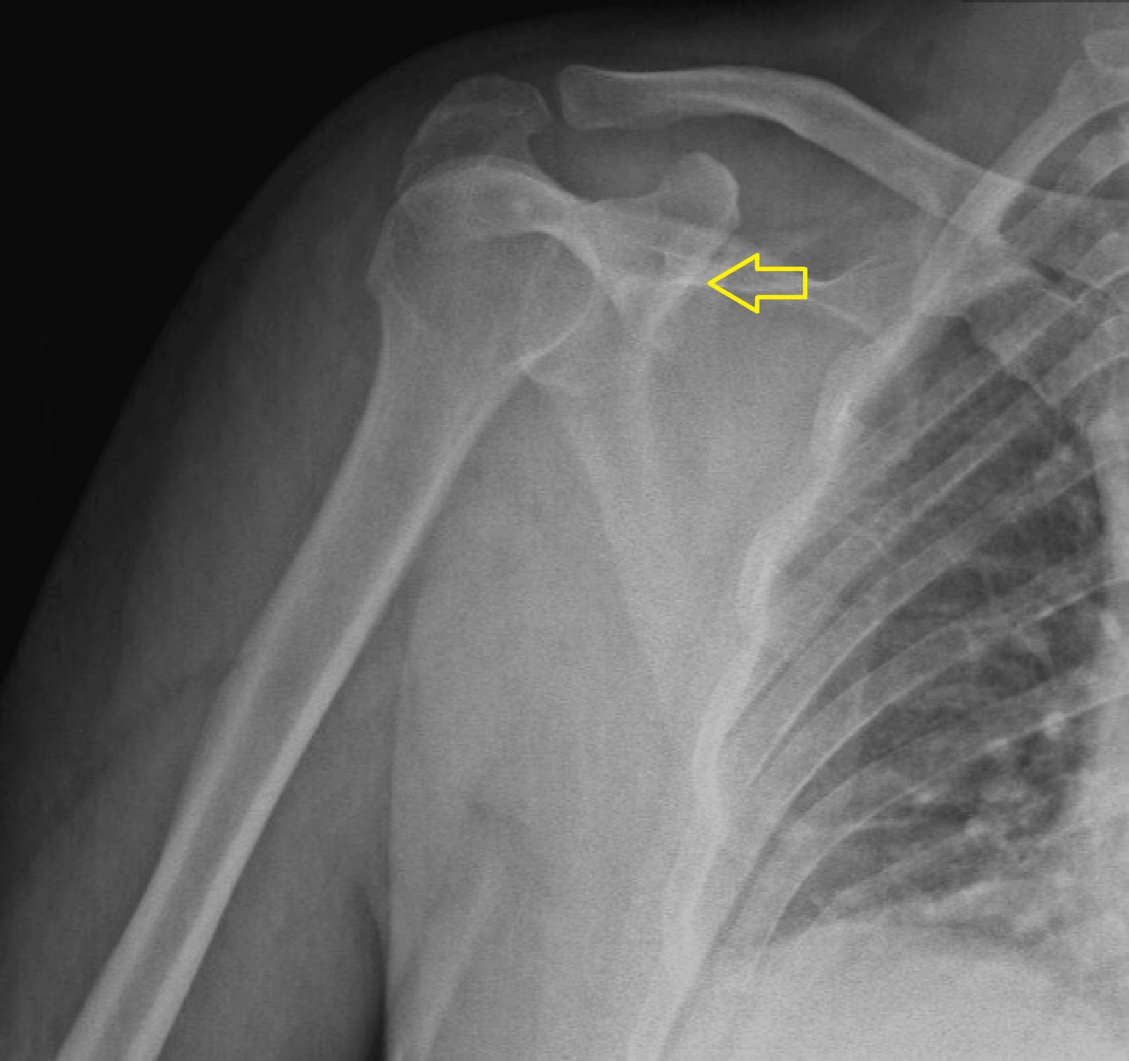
Preoperative X-ray of the right shoulder joint showing suspicion of a coracoid process fracture (yellow arrow).
